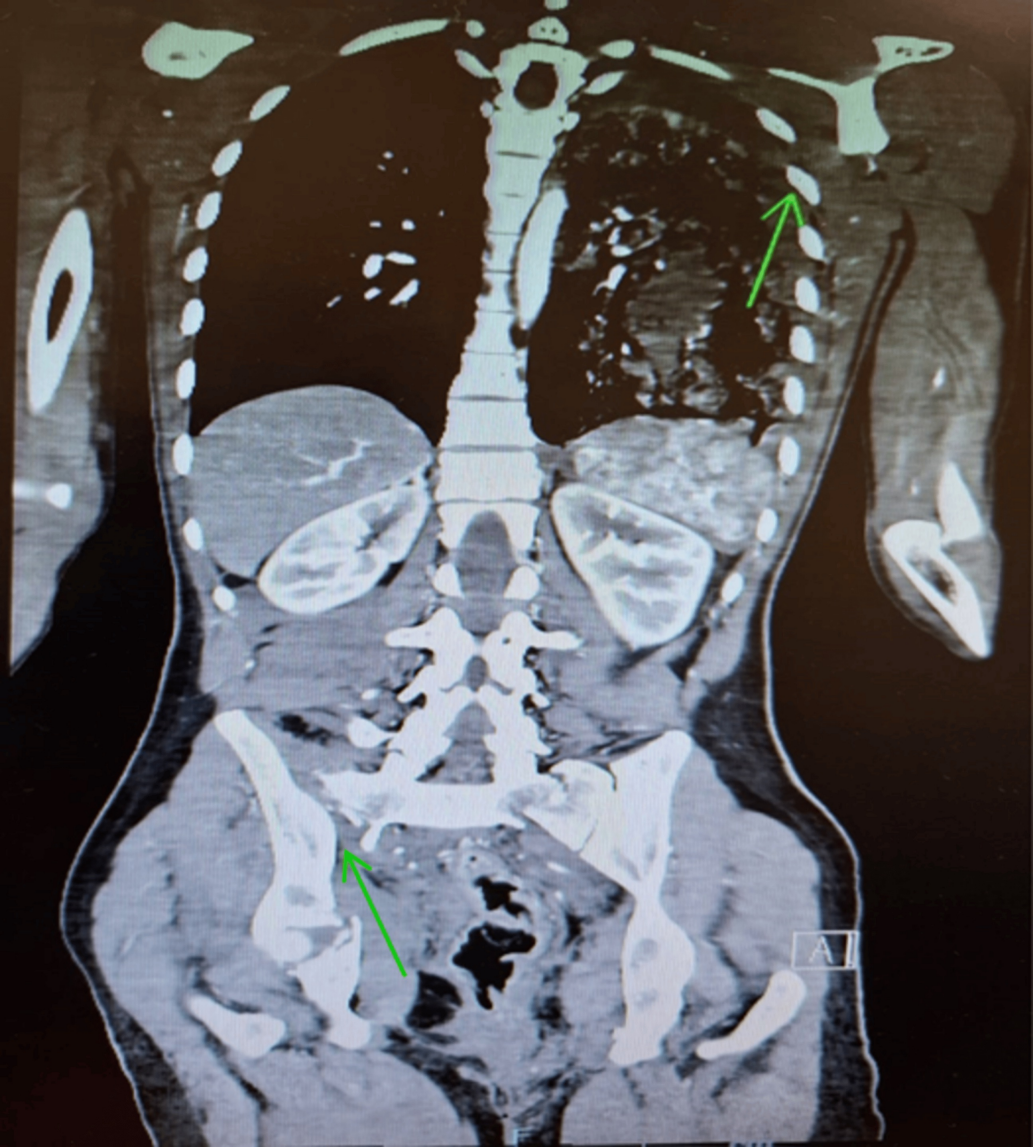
Contrast-enhanced CT demonstrating left hemo-pneumothorax, bilateral pulmonary contusions with intraparenchymal hemorrhage, left rib fractures, and complex pelvic and sacral fractures.
